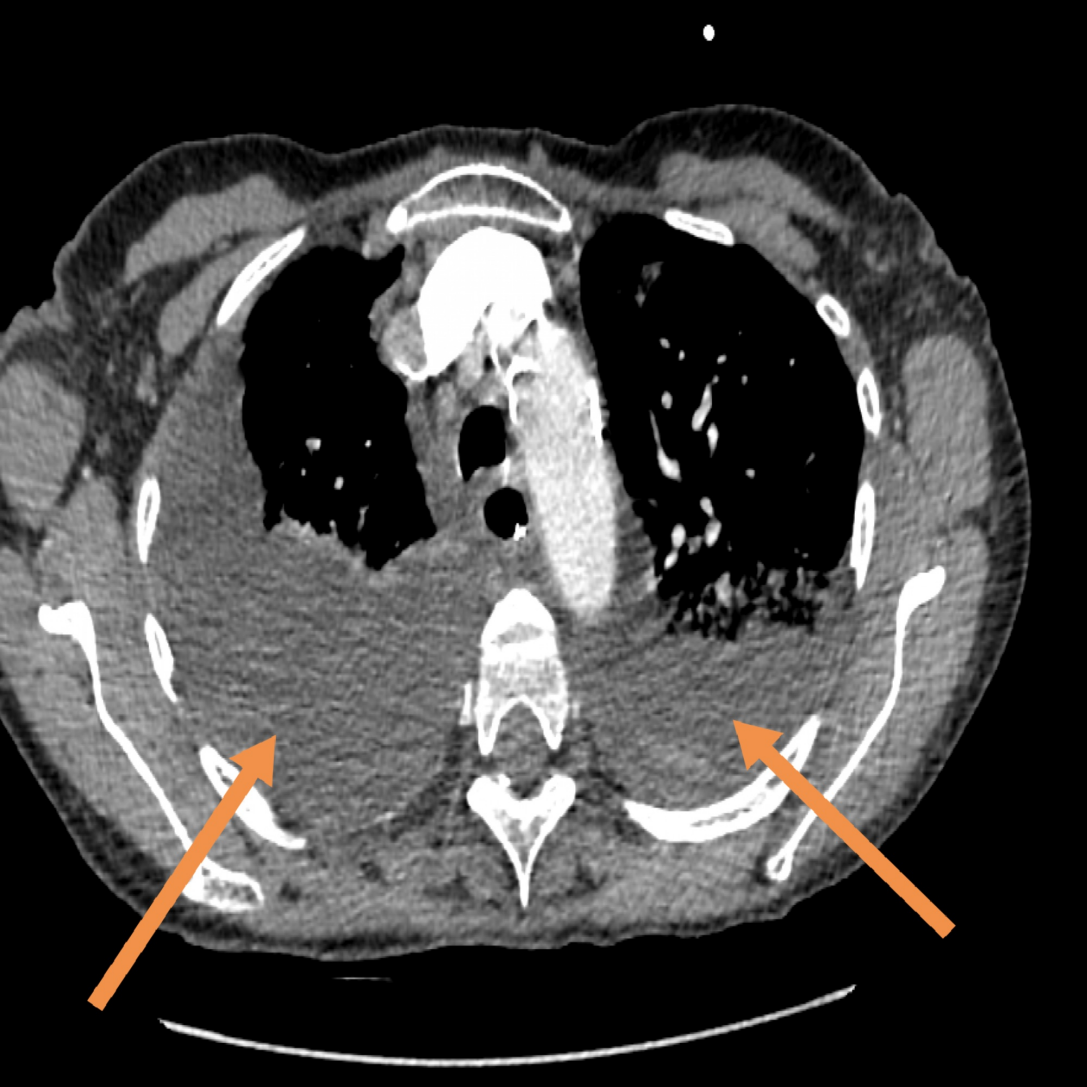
CT of the thorax showing bilateral empyema (see arrows)
